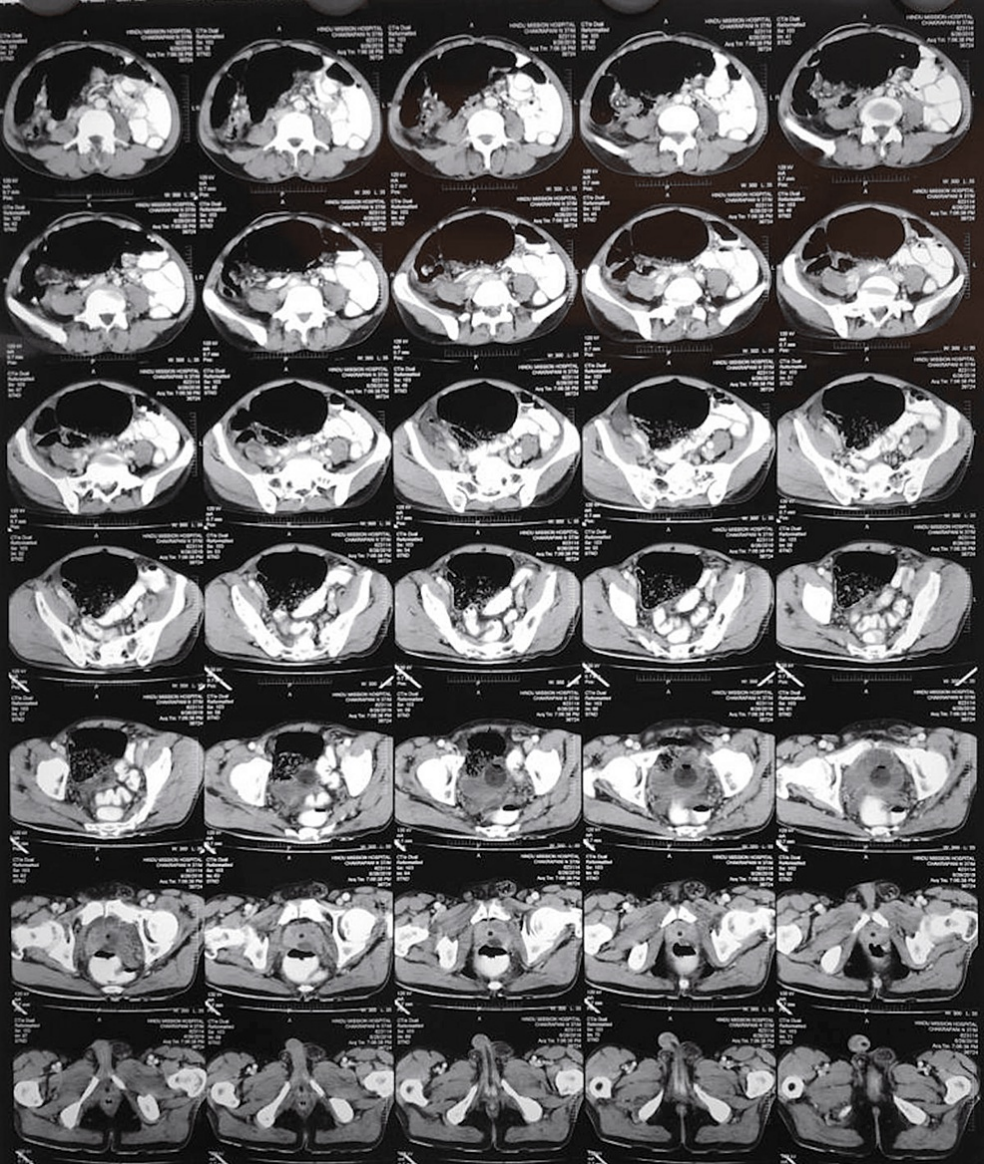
Preoperative abdominal CT image of the patient in favour of caecal volvulus. Gross dilatation of the caecum with a convergence of its two limbs was noted along with proximal dilatation of bowel loops. Mesenteric vessels are seen converging towards the dilated caecum with adjacent mesenteric fat stranding.
